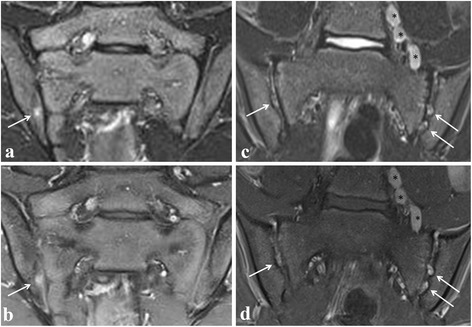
Active sacroiliitis in a 14-year-old (left) and 13-year-old (right) boy with juvenile spondyloarthritis according to a global assessment of MRI for sacroiliitis, not according to the ASAS definition of a positive MRI for sacroiliitis. Semicoronal STIR (a and c) and contrast-enhanced fat-saturated T1-weighted (b and d) images showing on the left side a focal enhancing BME lesion (seen on only one slice) at the iliac side of the right sacroiliac joint (arrows), and on the right side showing bilateral multiple enhancing spots of nodular high signal in the joint space, representing active erosions (arrows). No BME is seen. Note also the enlarged para-iliacal lymph nodes (asterisks)
